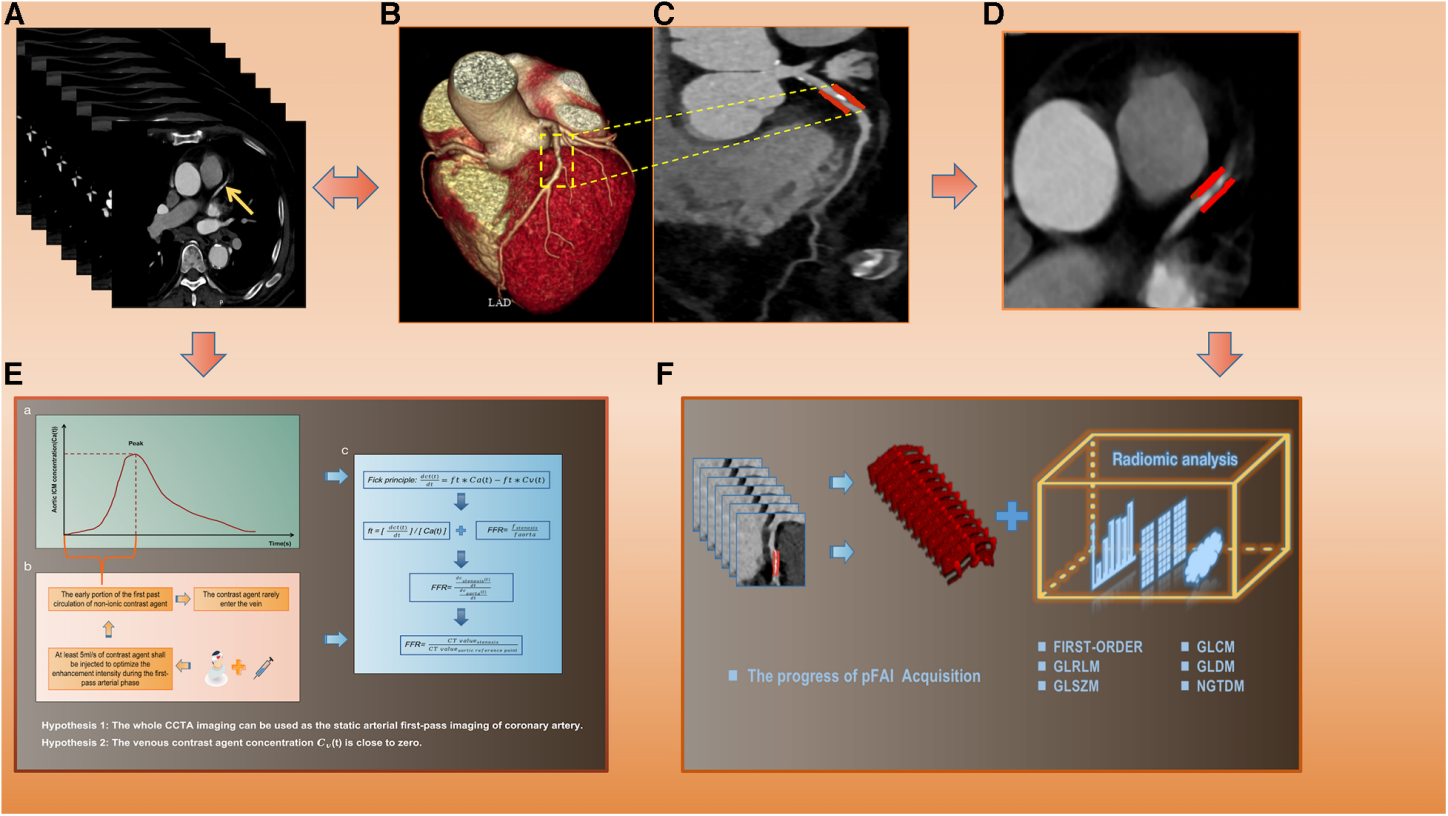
The specific calculation process of CT-FFR and pFAI and radiomics extraction. (A) Original CCTA images. (B,C) CCTA reconstruction images and lesion segments display. (D) PCAT ROI. (E) The calculation process and formula of CT-FFR. (F) pFAI acquisition and radiomics features analysis. CT-FFR, CT fractional flow reserve; pFAI, percoronary fat attenuation index; CCTA, coronary computed tomography angiography; PCAT, pericoronary adipose tissue.
